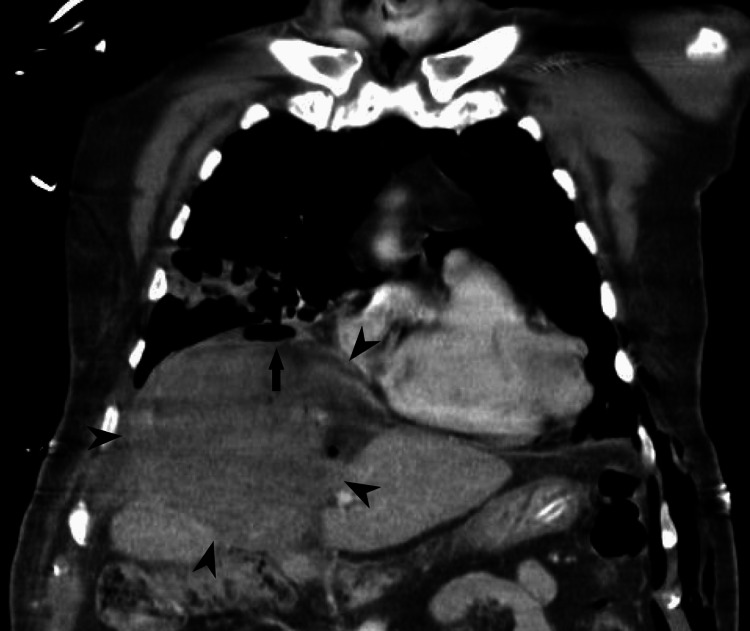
Coronal CT scan of the patient's thorax and upper abdomen. The image shows a large nodular formation in the upper region of the liver, measuring 110 mm in width, 134 mm in length, and 105 mm in height, with contrast-enhancing walls and an air-fluid level, suggestive of a hepatic abscess, marked by arrowheads. Continuity between the abdominal lesion and the pulmonary necrotizing process is noted, with diaphragmatic discontinuity, indicating diaphragmatic perforation secondary to the infectious process, marked by an arrow.
